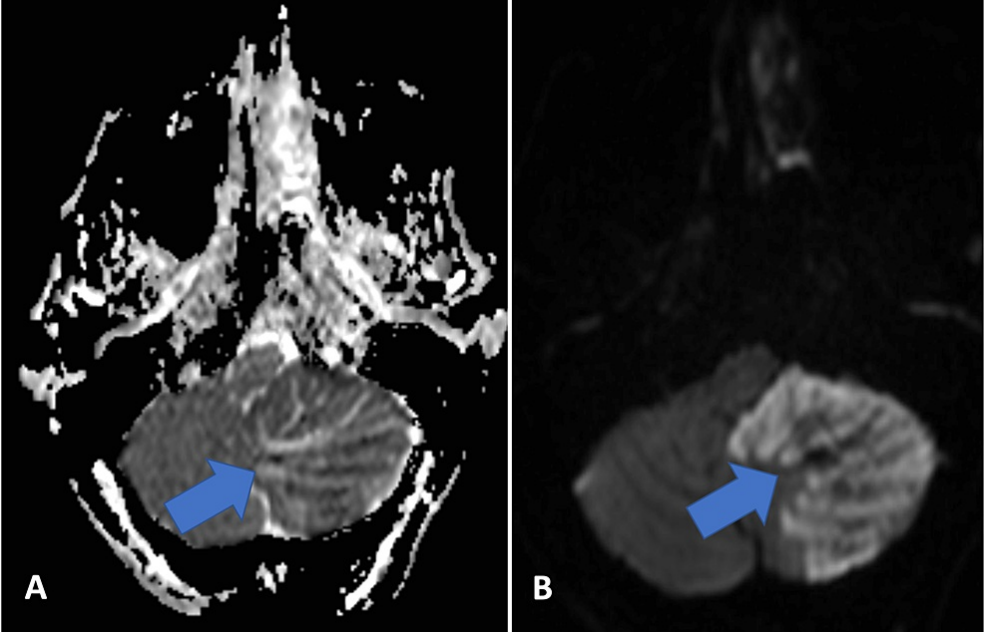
Axial apparent diffusion coefficient (ADC) map (A) and diffusion-weighted imaging (DWI) image (B) reveal striated/laminated appearance in the left cerebellum without significant diffuse diffusion restriction. The cerebellar cortex show hyperintensity most likely due to T2 shine-through effect.
